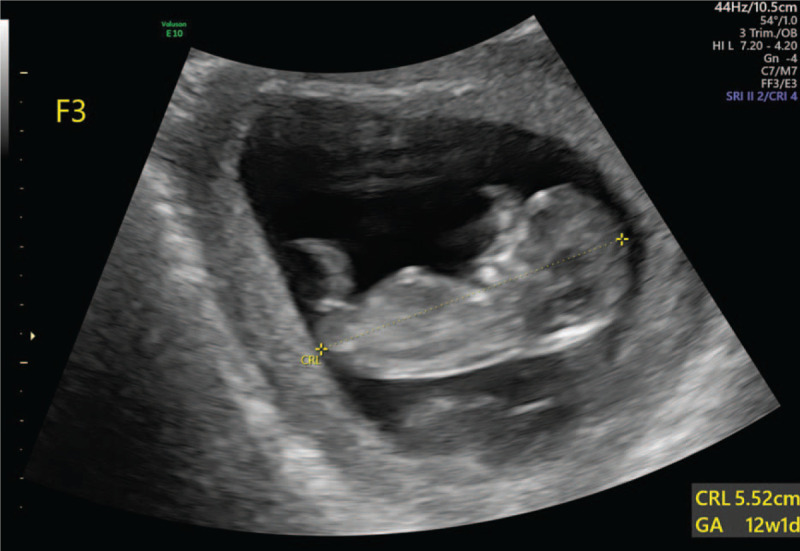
Two-dimensional ultrasound image of the normal nonconjoined fetus.
